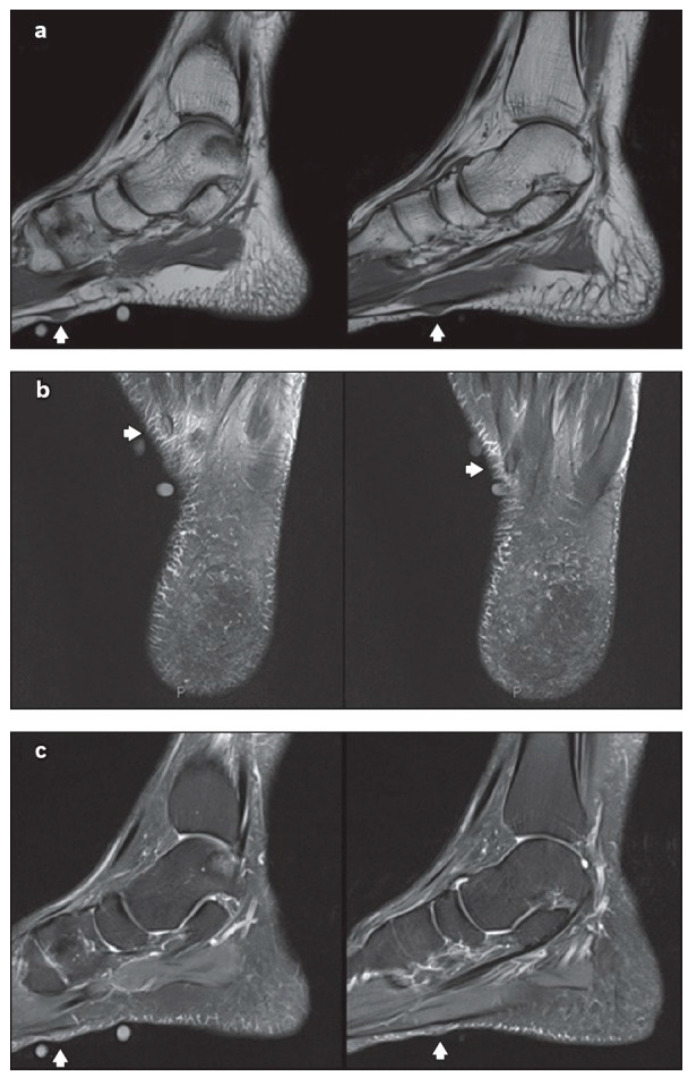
Non-contrast-enhanced magnetic resonance (MR) imaging showing two sub-centimetre nodules (arrows of a–c) along the inner band of the plantar fascia, on the left foot: (a) sagittal T1-W, (b) axial T2-W fat saturation and (c) sagittal proton density-weighted fat saturation MR images of the left foot. Source: Reprinted from Teo, F.; Mohamed Shah, M.T.; Wong. Clinics in diagnostic imaging. *Singapore Med. J.* 2019 [37] (Singapore Med. J. licensed under CC BY-NC-SA 4.0, no permission required).
